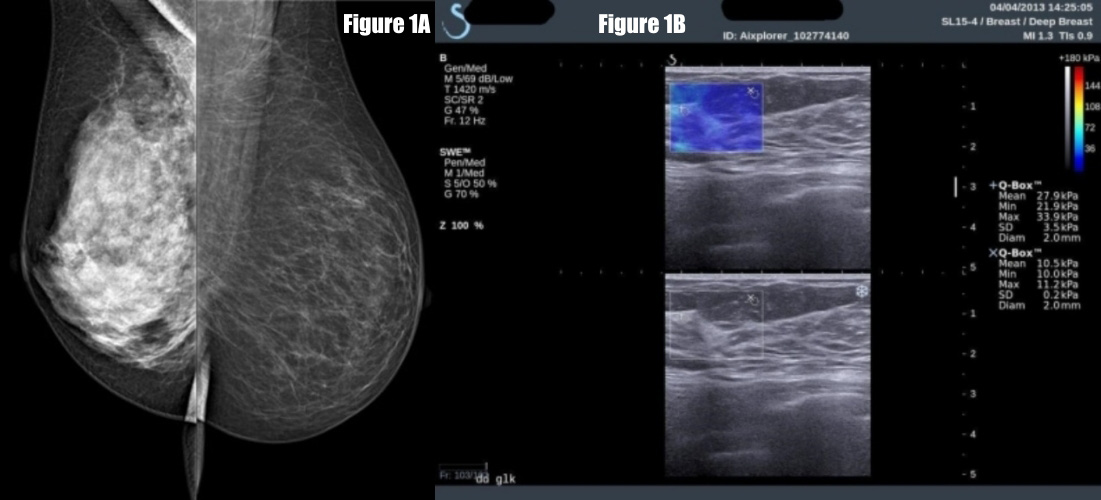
(A) Different breast densities on mammography: left – extremely dense breast; right – entirely fatty breast. (B) Sonoelastographic measurement showing heterogeneous breast density. The region of interest is placed in the representative area of parenchymal and adipose tissue. Ultrasound device shows elasticity values in color and kilopascals.
